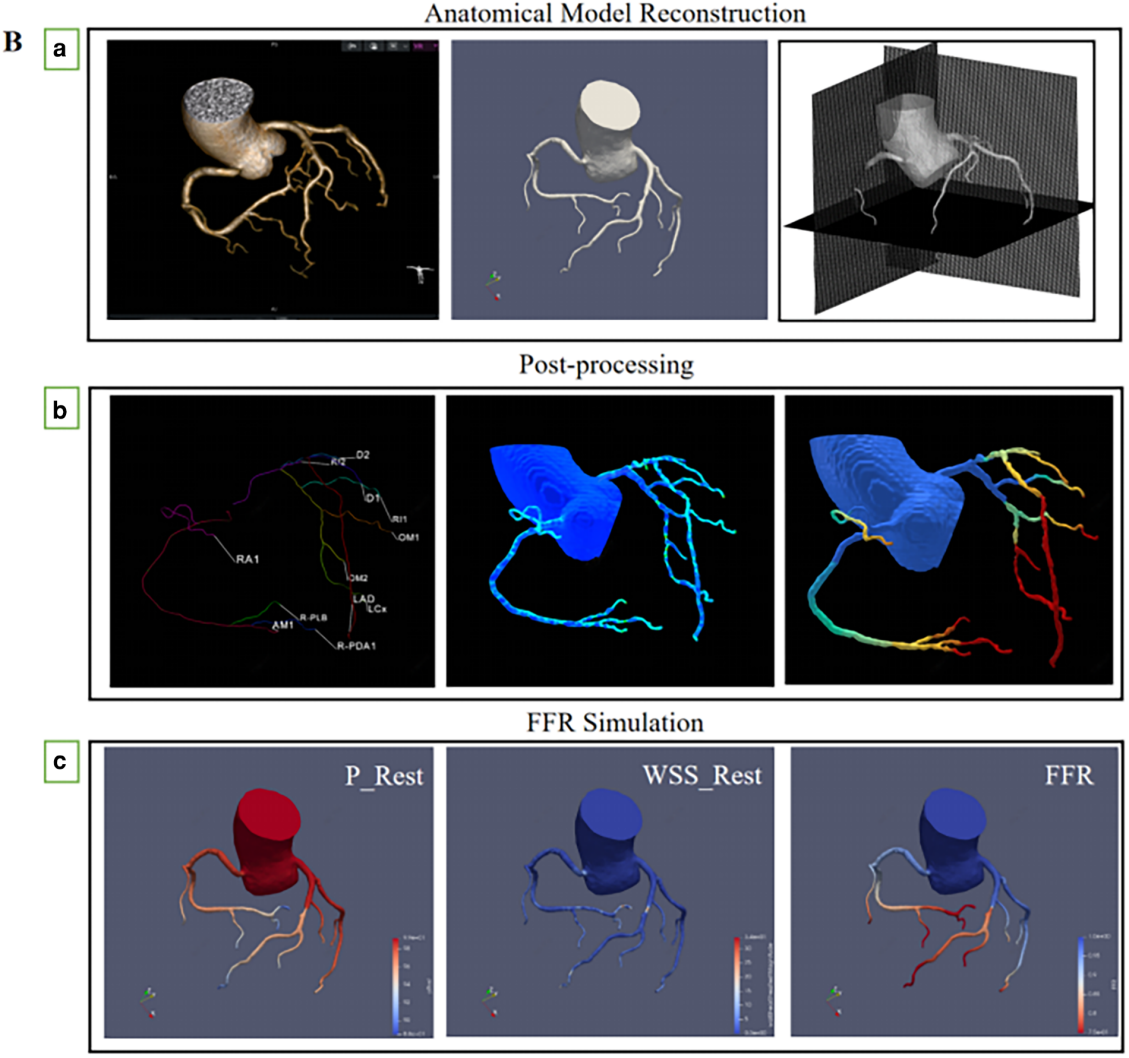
(A) multiplanar reformatting of (a) coronary CT angiography, (b) CT-FFR of the right coronary artery system, and CT-FFR value (0.886) was measured (c) invasive coronary angiography, (d) invasive FFR measurements, the FFR measured at the corresponding position was 0.88. (B) (a) Routine coronary computed tomography angiography are received and reconstruction of anatomical model, (b) Coronary artery physiological models were measured at corresponding locations, (c) The physical laws of fluid dynamics are used to calculate coronary blood flow, and calculation of fractional flow reserve from a standard acquired coronary computed tomography datasets.
